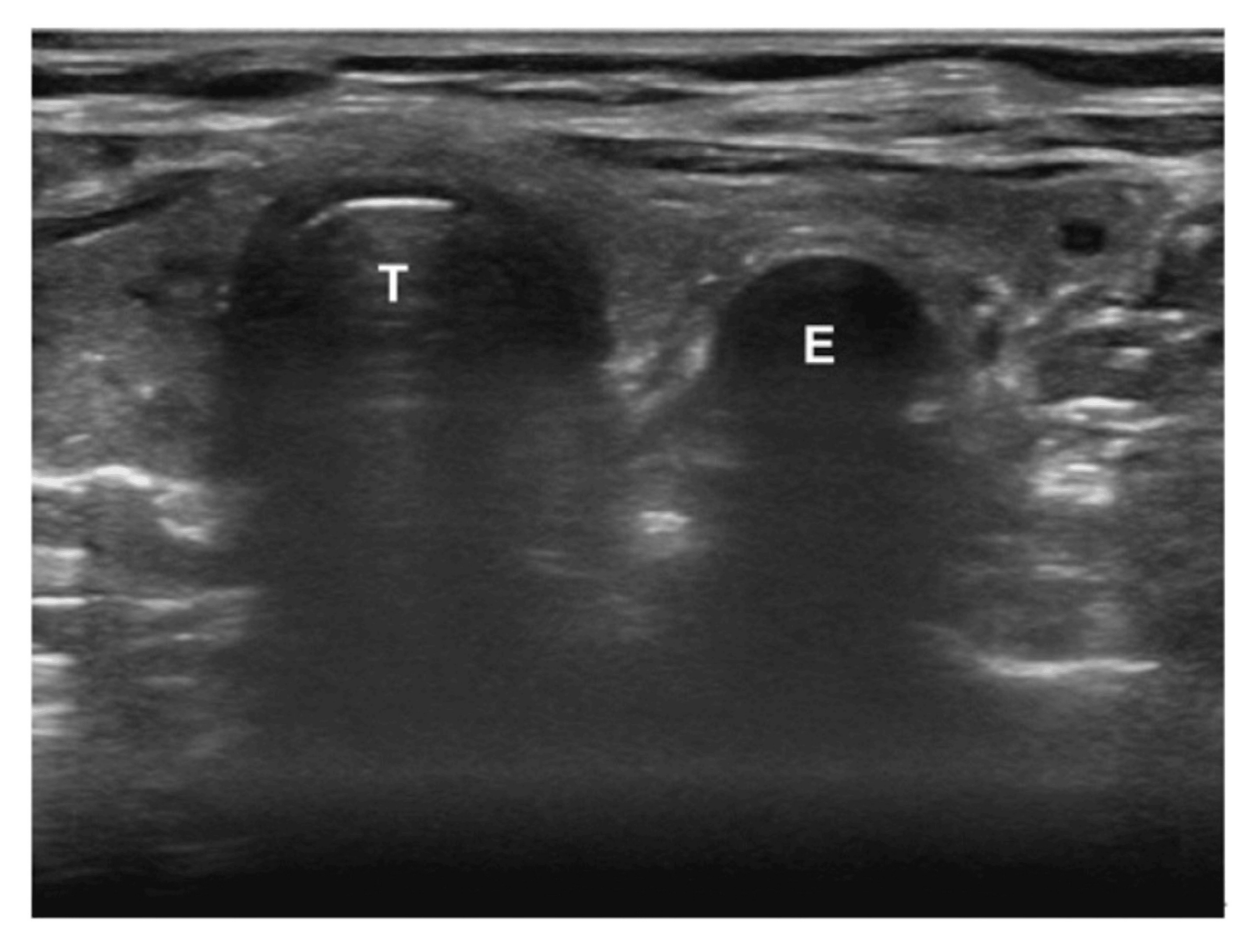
Airway ultrasound showing esophageal intubation In the case of esophageal intubation, a curvilinear structure that mimics the trachea (T) is seen to the right of the trachea. This is the ETT within the esophagus (E) [35]. ETT: endotracheal tube Gottlieb et al. (2024) [35]; Creative Commons Attribution (CC BY) license
